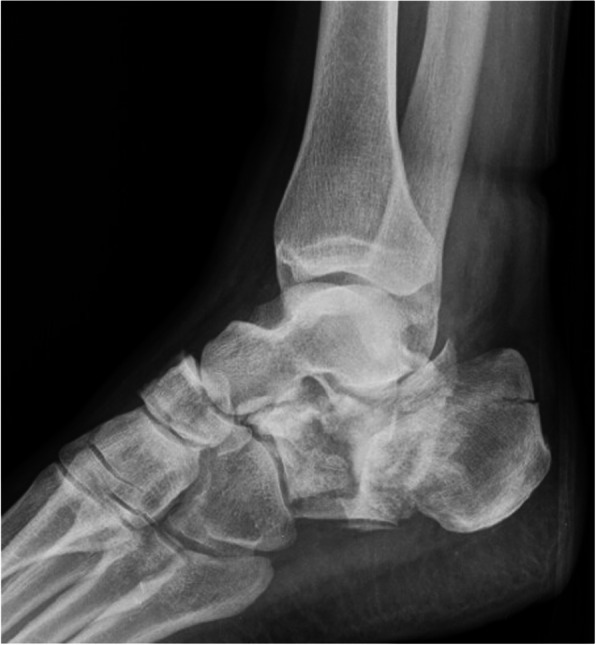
Case 2 Preoperative radiographs. Radiograph right calcaneus lateral, 35 year old female patient, smoker
